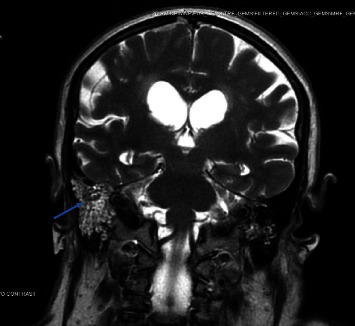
MRI of the brain (case 1) of a 75-year-old female right mastoid effusion with enhancement. Demonstrated by the blue arrow.
